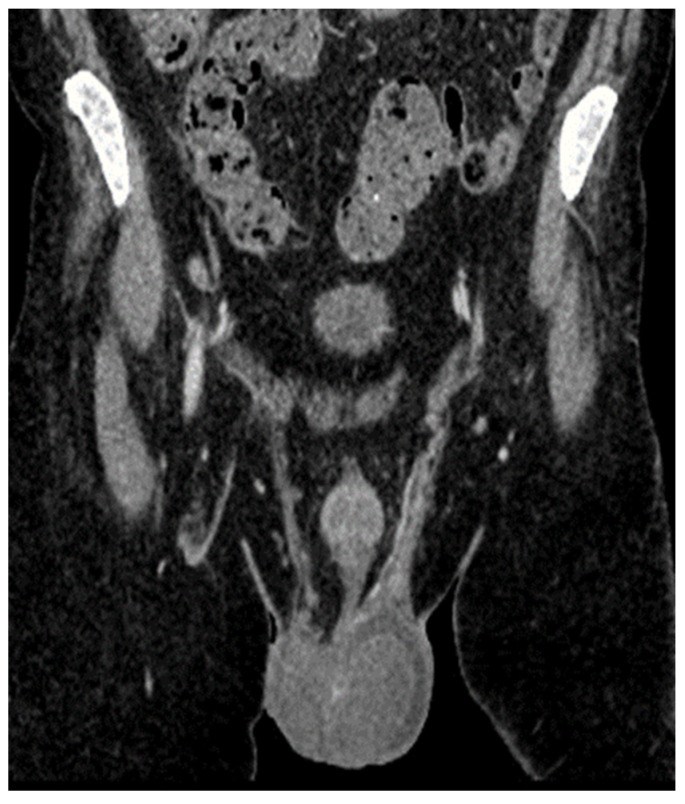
Comparative abdomino-pelvic CT examination—dimensionally reduced left testicle.
